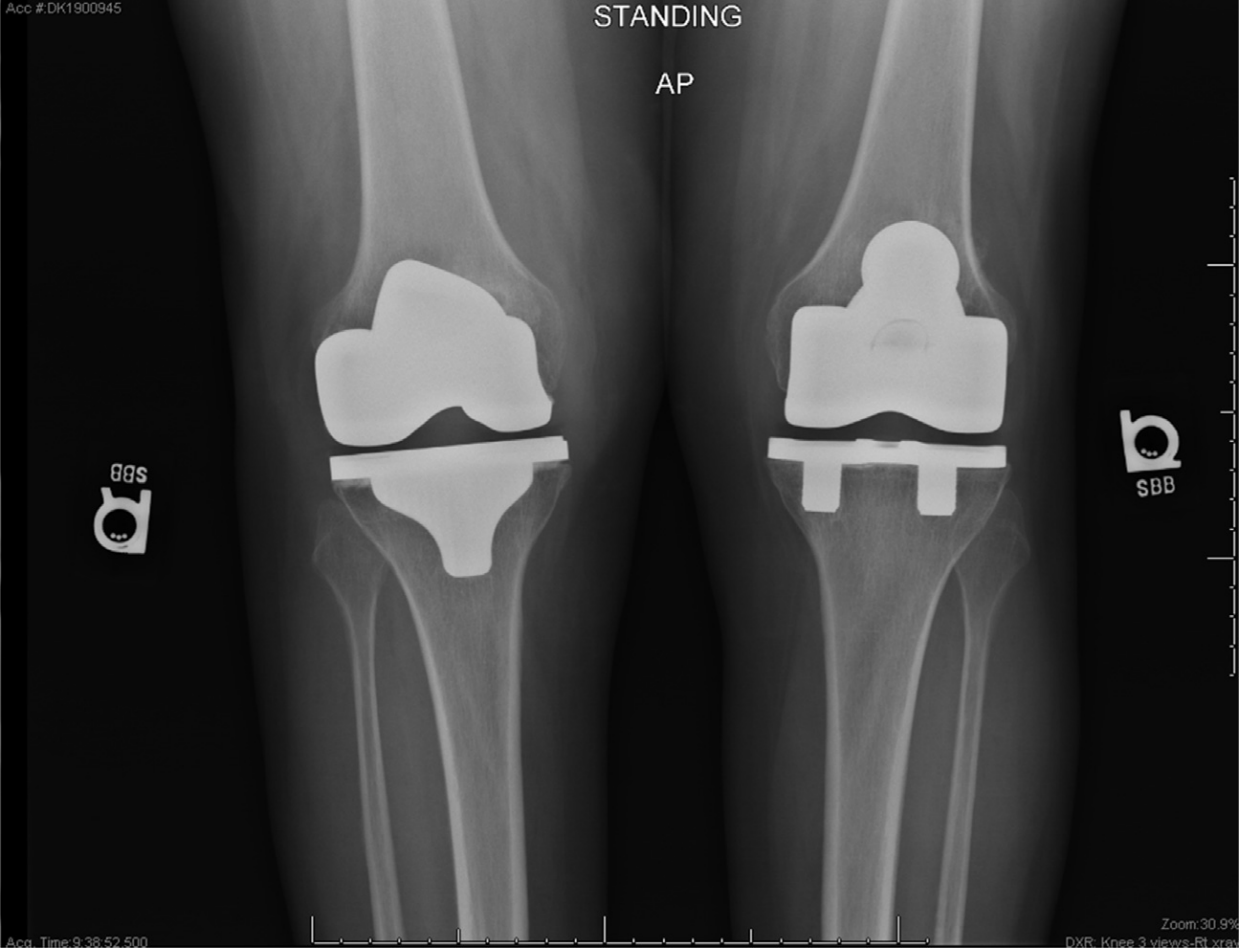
Standing anteroposterior radiograph 8 months after operation with no evidence of tibial baseplate fracture.
